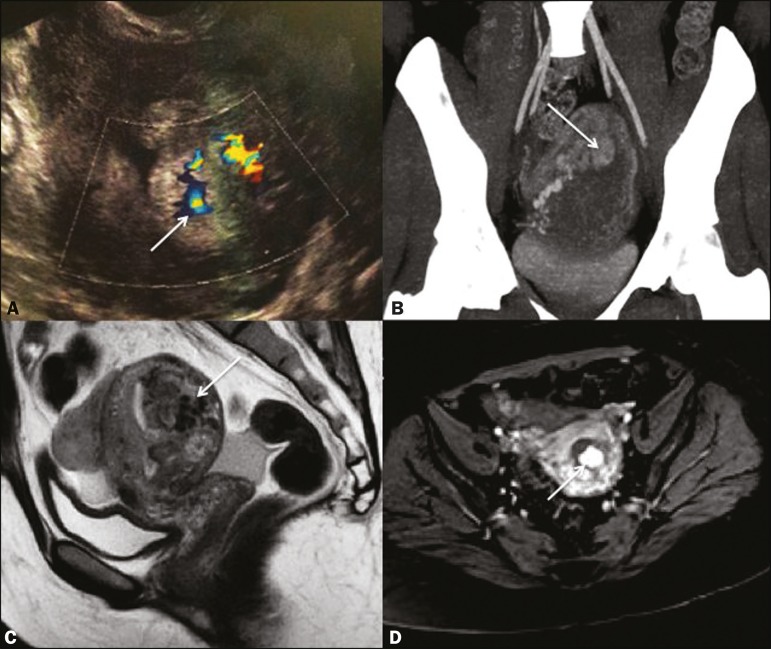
A: Transvaginal ultrasound showing a heterogeneous endometrial
